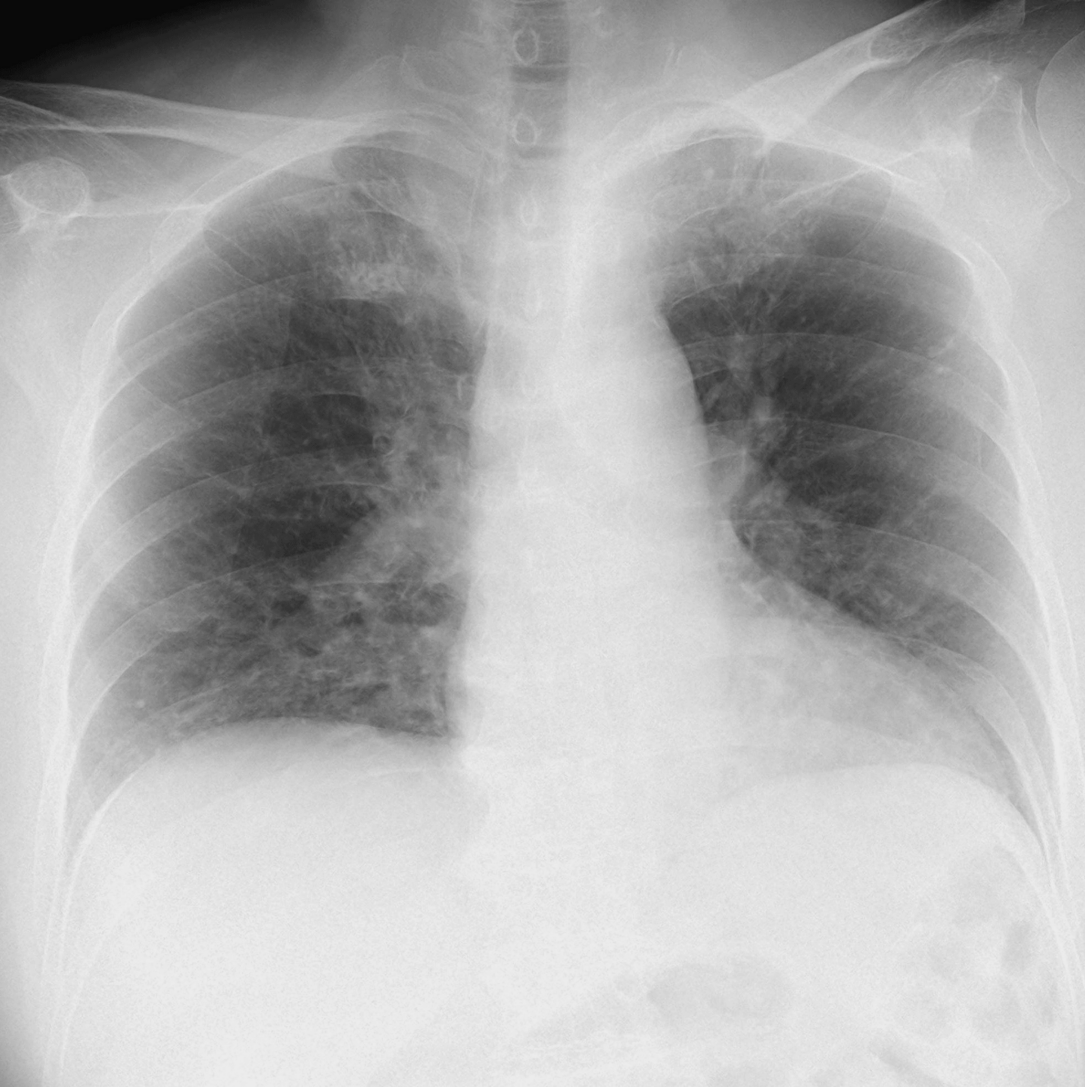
Chest X-ray in the sitting position showed no evidence of active pulmonary lesions.
